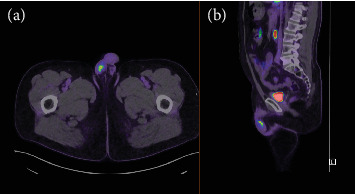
PSMA PET. Axial slice (a). Sagittal slice (b). Avid region correlates with right testis.
